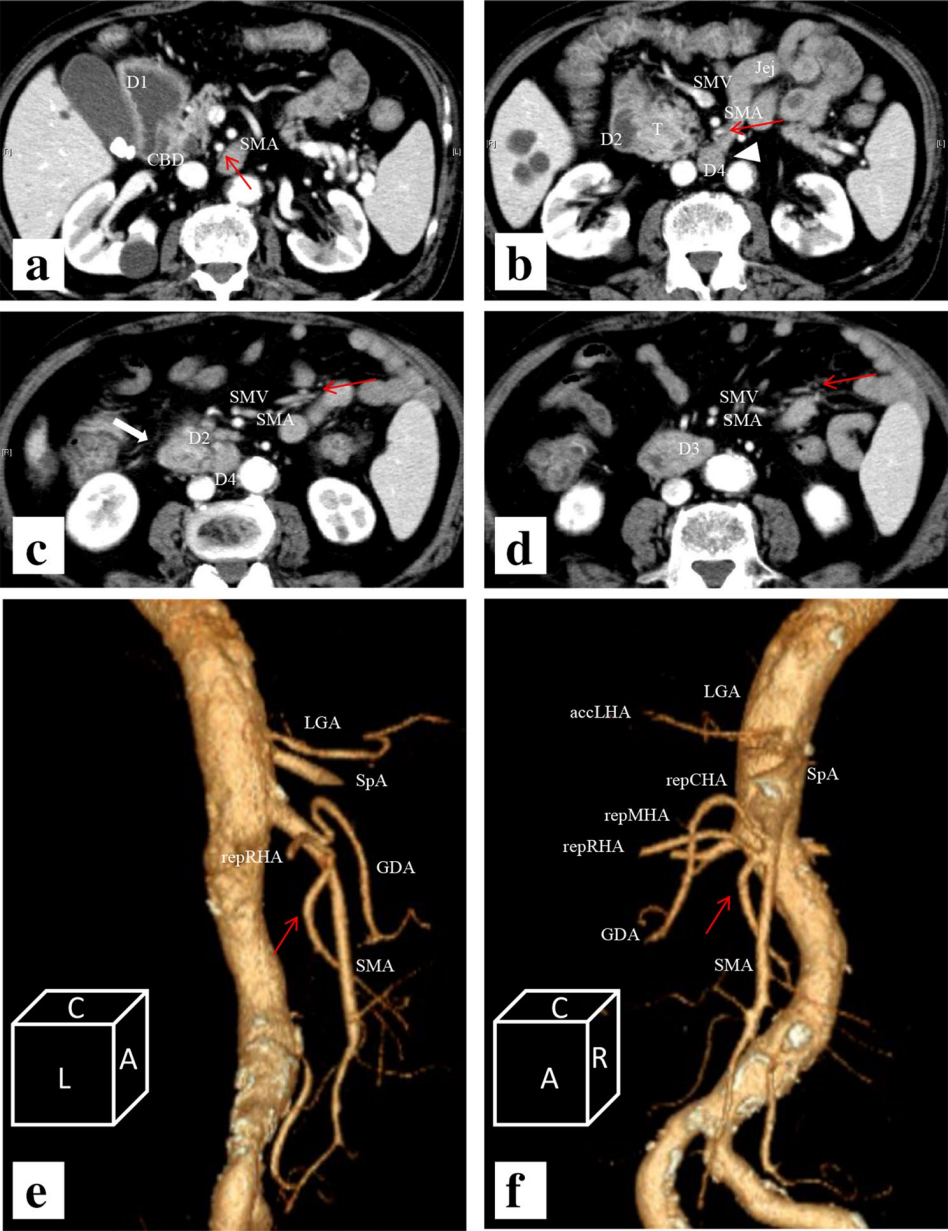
Contrast-enhanced computed tomography (CT) a showed a dilated first portion of the duodenum and an intact intra-pancreatic common bile duct. Both the higher replaced first jejunal artery (repFJA) (red arrow) and superior mesenteric artery (SMA) were similar in diameter, i.e. 7 and 9 mm, respectively, thereby, resembling duplicated SMAs. b Low-density lesion invading the second portion of the duodenum (D2), but not involving the repFJA (red arrow), SMA or superior mesenteric vein (SMV). In addition, the transposition of the fourth portion of the duodenum (D4) and jejunum did not reach the left side of the aorta, but stopped at the right side of it (arrowhead). c CT demonstrated D2, D4, repFJA (red arrow), SMA and SMV. Soft-density tissue (thick arrow) surrounding D2 might be the part of annular pancreas located at the caudal side of the pancreatic head tumour. d CT displayed repFJA (red arrow), SMA, SMV and a very short third portion of the duodenum. e Reconstructed CT angiography in the left lateral position depicted three arteries arising from the aorta namely, the left gastric artery (LGA) bearing the accessory left hepatic artery (accLHA), splenic artery (SpA) and SMA. The replaced common hepatic artery (repCHA), from which the gastroduodenal artery (GDA) and replaced middle hepatic artery (repMHA) arose, and a common trunk of the replaced right hepatic artery (repRHA) and higher repFJA (red arrow) were, respectively, bifurcated near the root of the SMA. f Reconstructed CT angiography in the right anterior oblique added for the comprehension of the complicated arterial variations as described. *AccLHA* accessory left hepatic artery, *CBD* common bile duct, *D1* first portion of the duodenum, *D2* second portion of the duodenum, *D3* third portion of the duodenum, *D4* fourth portion of the duodenum, *GDA* gastroduodenal artery, *Jej* jejunum, *LGA* left gastric artery, *PV* portal vein, *repCHA* replaced common hepatic artery, *repMHA* replaced middle hepatic artery, *repRHA* replaced right hepatic artery, *SpA* splenic artery, *SMA* superior mesenteric artery, *SMV* superior mesenteric vein, *T* tumour
